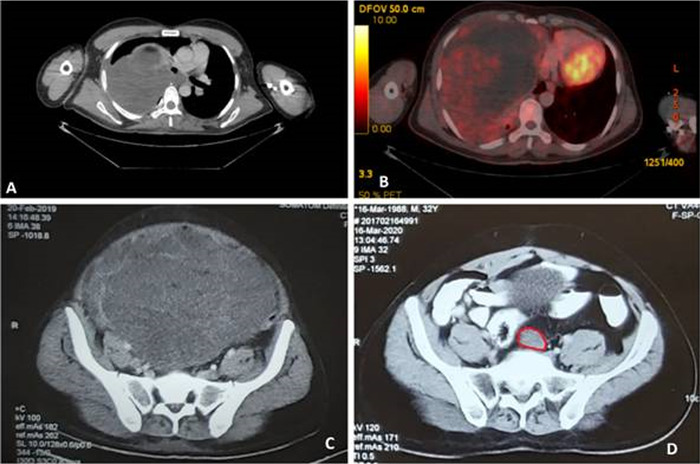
(A) Contrast-enhanced computed tomography (CECT) shows pleural-based heterogenous hypodense mass. (B) Baseline positron emission tomography–computed tomography shows local disease. (C) CECT shows large abdominopelvic mass. (D) CECT shows presacral deposit.
